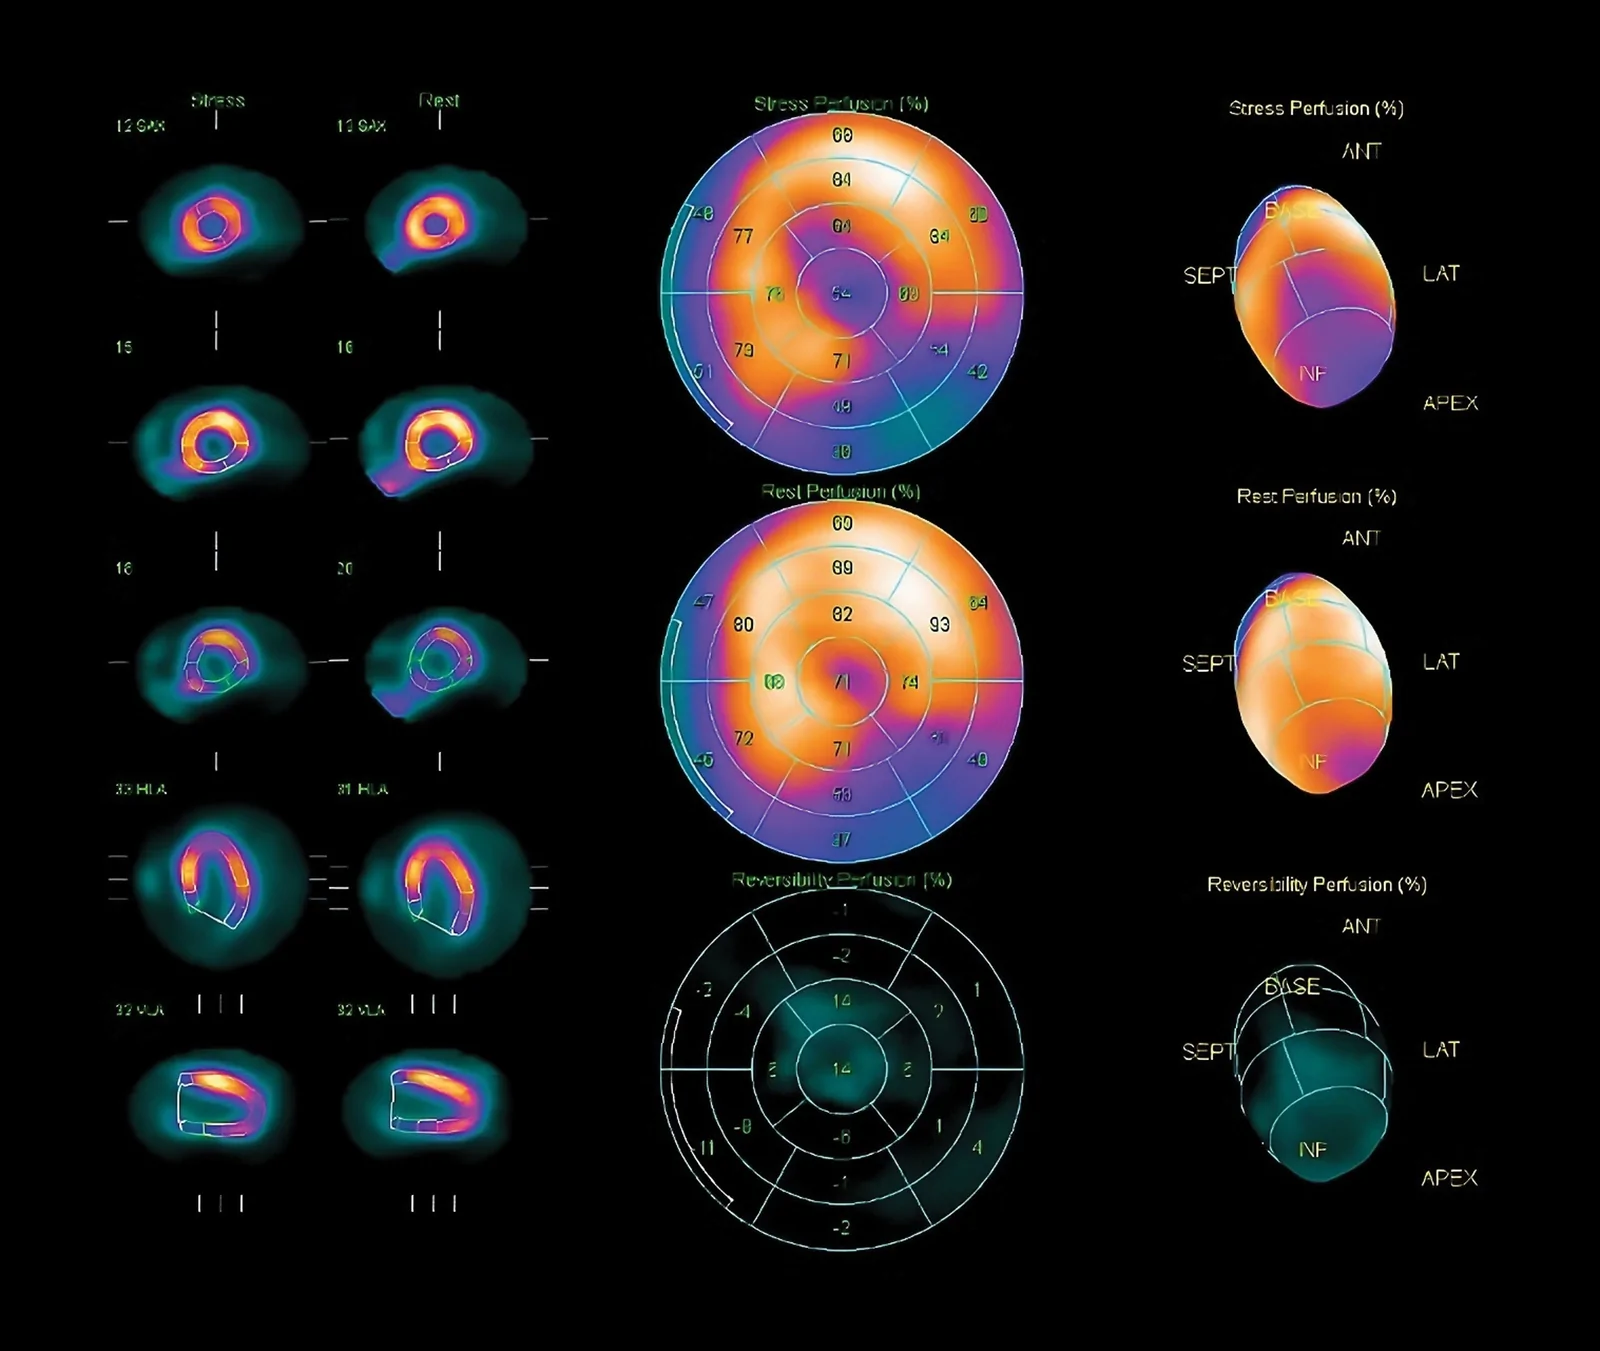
Post-operative stress-rest myocardial perfusion scintigraphy demonstrating persistent hypoperfusion of the inferolateral and apical regions, unchanged from the pre-operative study.
